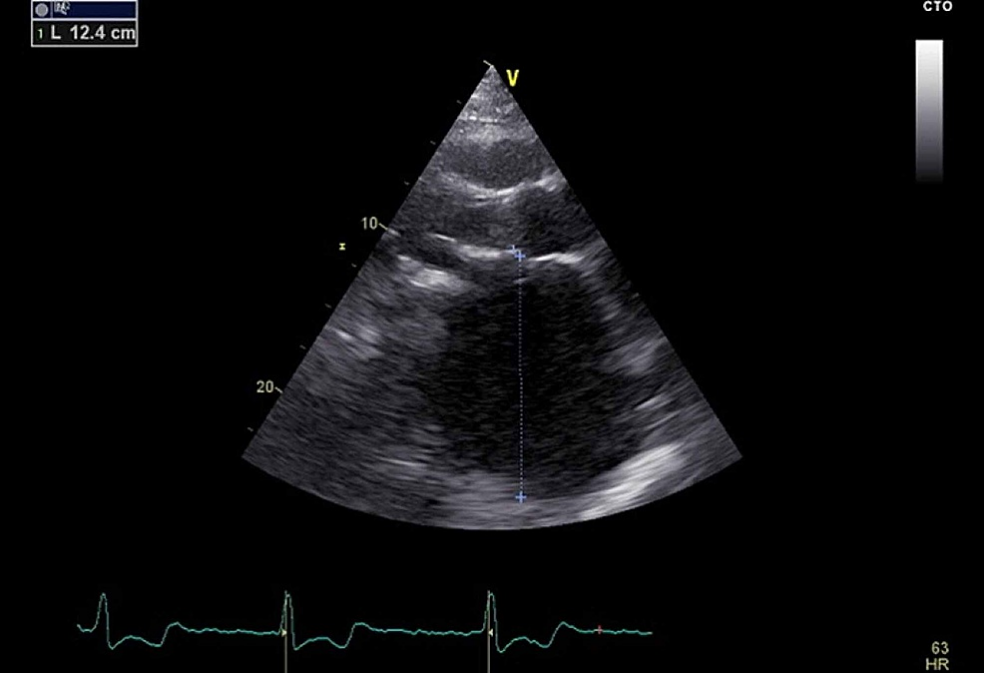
Echocardiography image of the left atrium (LA diameter) on the parasternal long-axis view
